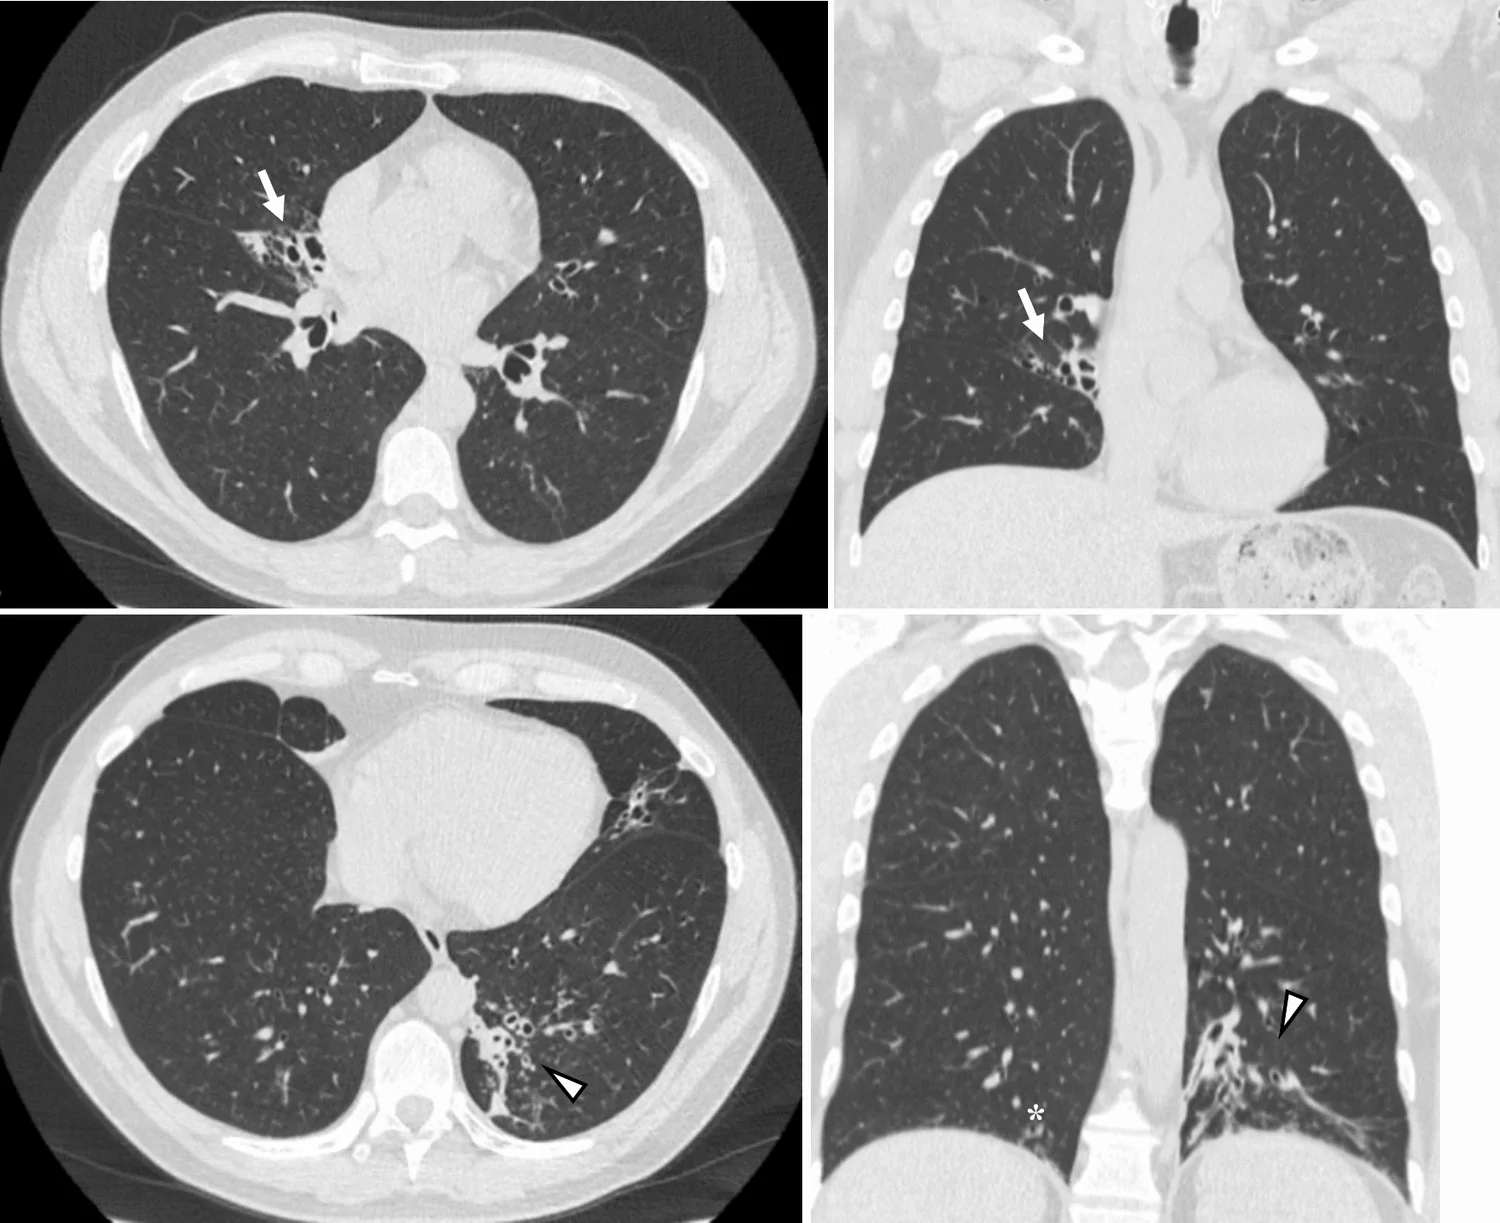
High-resolution chest computed tomography of the proband. Cylindrical and varicose bronchiectasis and endobronchial plugs are predominantly observed in the right middle lobe (arrows) and in the posterior-basal segments of the left lower lobe (arrowheads). Additionally, tree-in-bud micronodules (*) are present in the middle-right lobe and bilaterally in the lower lobes, suggestive of ongoing inflammatory/infectious processes with endobronchial dissemination
